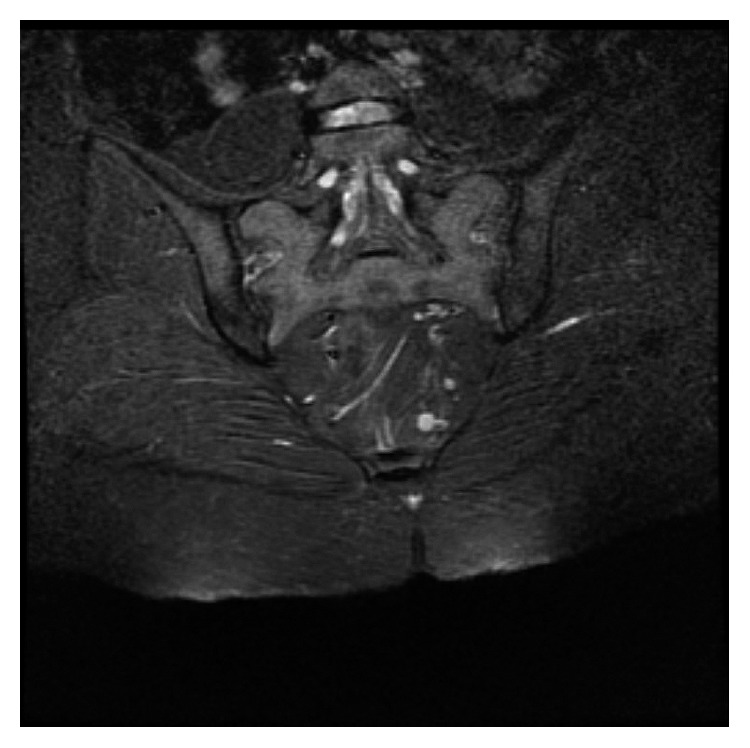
Coronal fat-suppressed T2-weighted MRI scan of the sacroiliac joints in favor of acute sacroiliitis and bilateral sclerosis.
